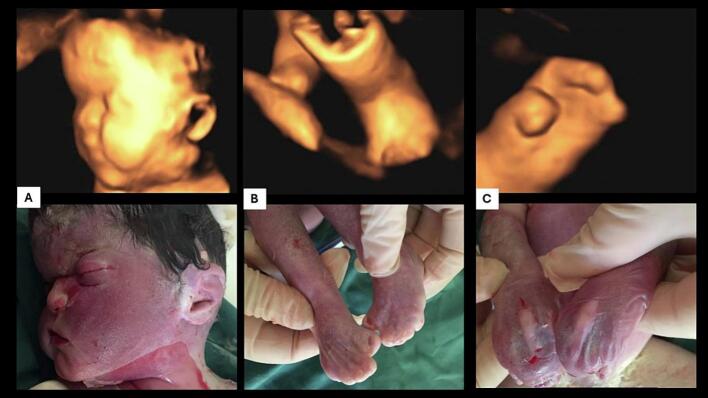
Comparison between 3D ultrasound findings and macroscopic fetal examination showing the same facial dysmorphia (A) and limb abnormalities (B, C).
